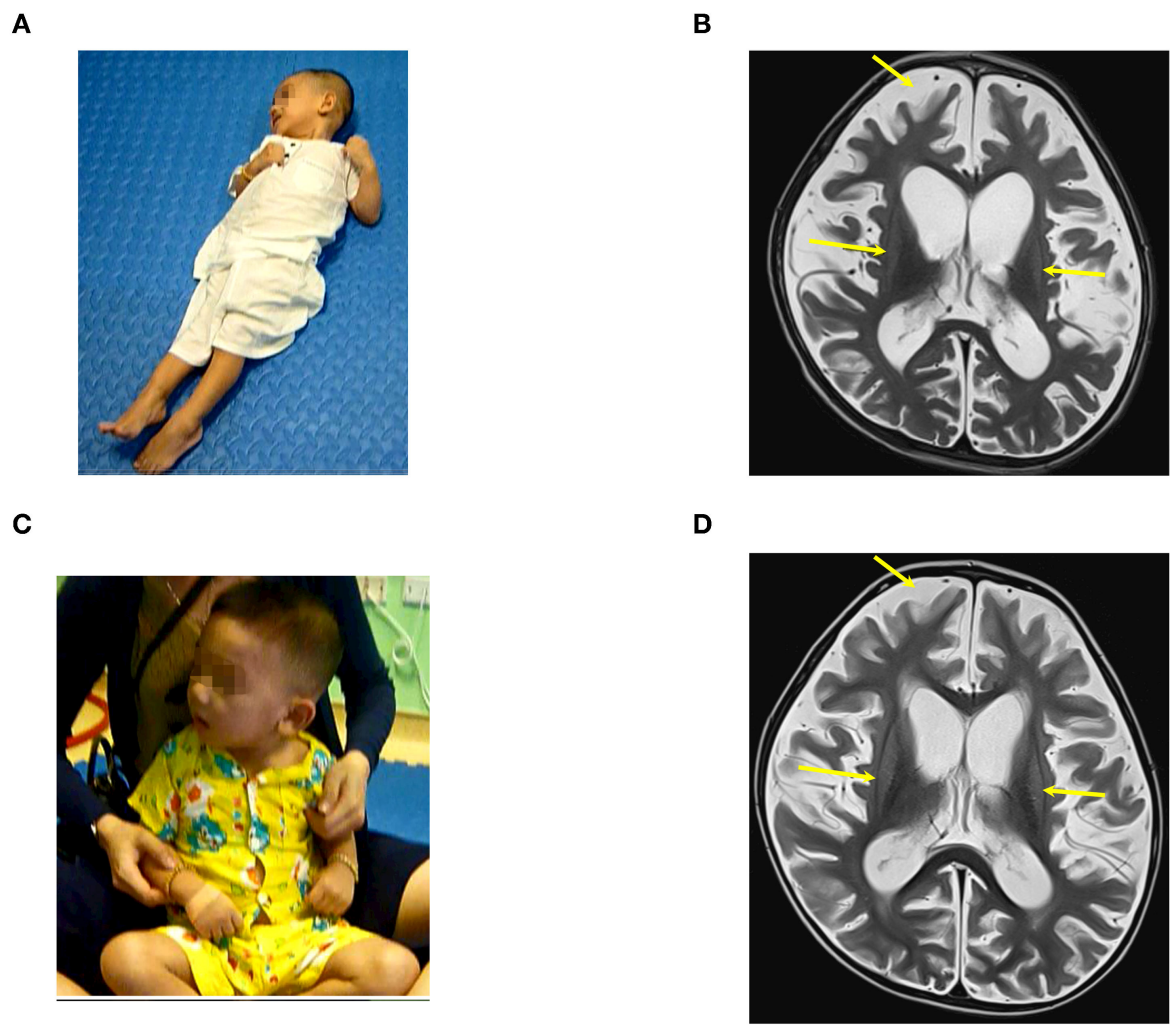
The patient (case 5) before and after autologous BMMNC transplantation; (A) 2.5 months after the drowning before BMMNC transplantation, the patient is in a VS. without awareness, no motor function, having tetraplegia, and exhibited muscle spasticity at level V. (B) Diffuse brain atrophy and abnormal signal at bilateral putamen on brain MRI before transplantation (arrows). (C) Six months after the second transplantation, the patient could display limited vocal capacity and turn and sit up with support. (D) There was no significant change in brain MRI after transplantations compared with before transplantation.
